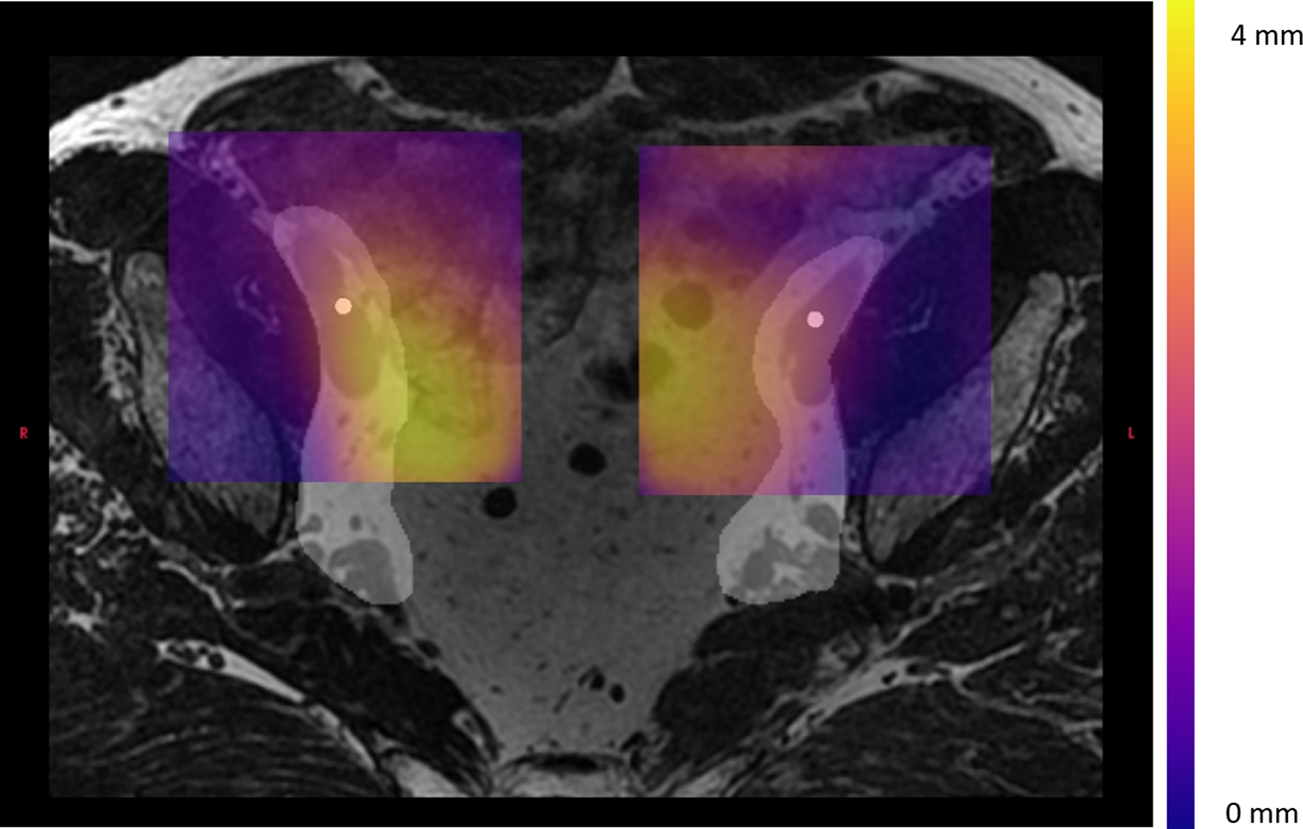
Vector fields showing deviation from baseline MRI in right anterior and left anterior in colors. The white structure is CTV and the white dot is COM
